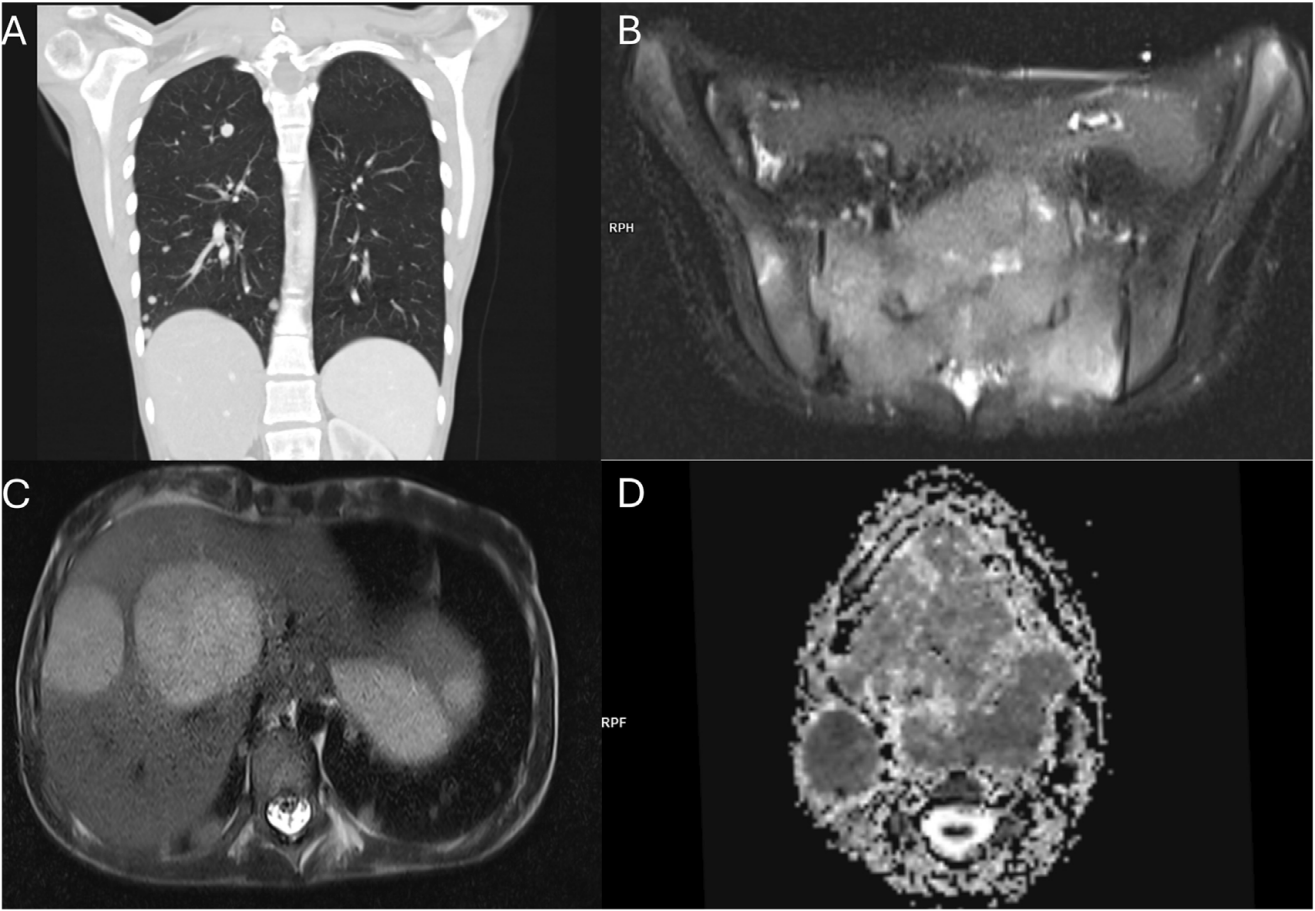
Staging imaging examinations at diagnosis of ASPS in 12.2023. (A) Coronal reconstruction of the lung CT scan demonstrating multiple metastatic lesions in the lung parenchyma. (B) T2-weighted MRI with fat saturation showing a sacral bone metastasis. (C) T2-weighted MRI revealing metastatic lesions in the liver. (D) MRI-apparent diffusion coefficient (ADC) map depicting the tongue mass and pathological cervical lymph nodes.
